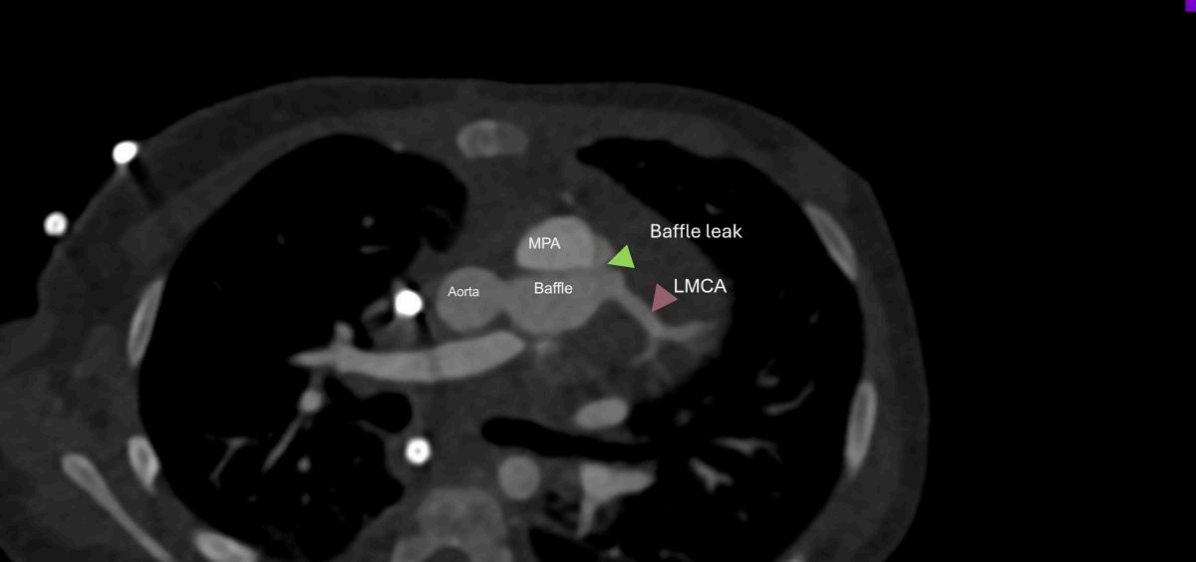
Pre-intervention computed tomography angiography. Post the Takeuchi repair, computed tomography angiography confirms a connection from the baffle into the main pulmonary artery, the diameter of the leak is about 2.2 mm (green arrow). Also seen is the LMCA (pink arrow).
